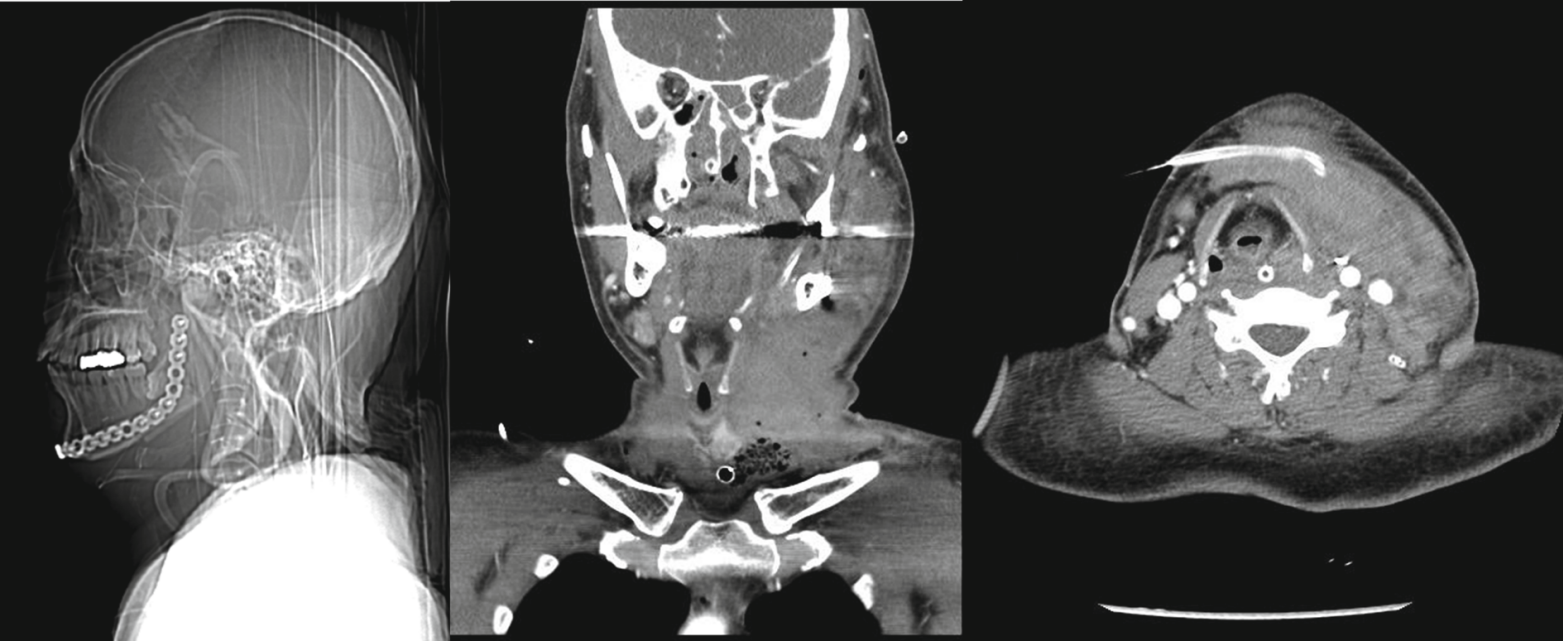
Neck computed tomography shows severe swelling and soft tissue density lesion in the left face and neck with airway compression.
